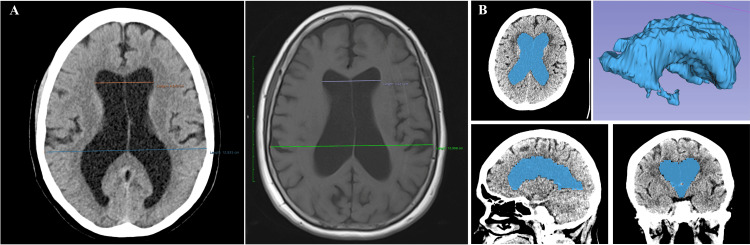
Axial image measuring the Evans index as the ratio between the frontal horns' maximum width and the cranium's maximum inner diameter on CT and T1-weighted images (A). CT image showing the segmentation of the ventricular volume shaded in blue on axial, sagittal, and coronal images with final segmented output (B). CT: computed tomography
